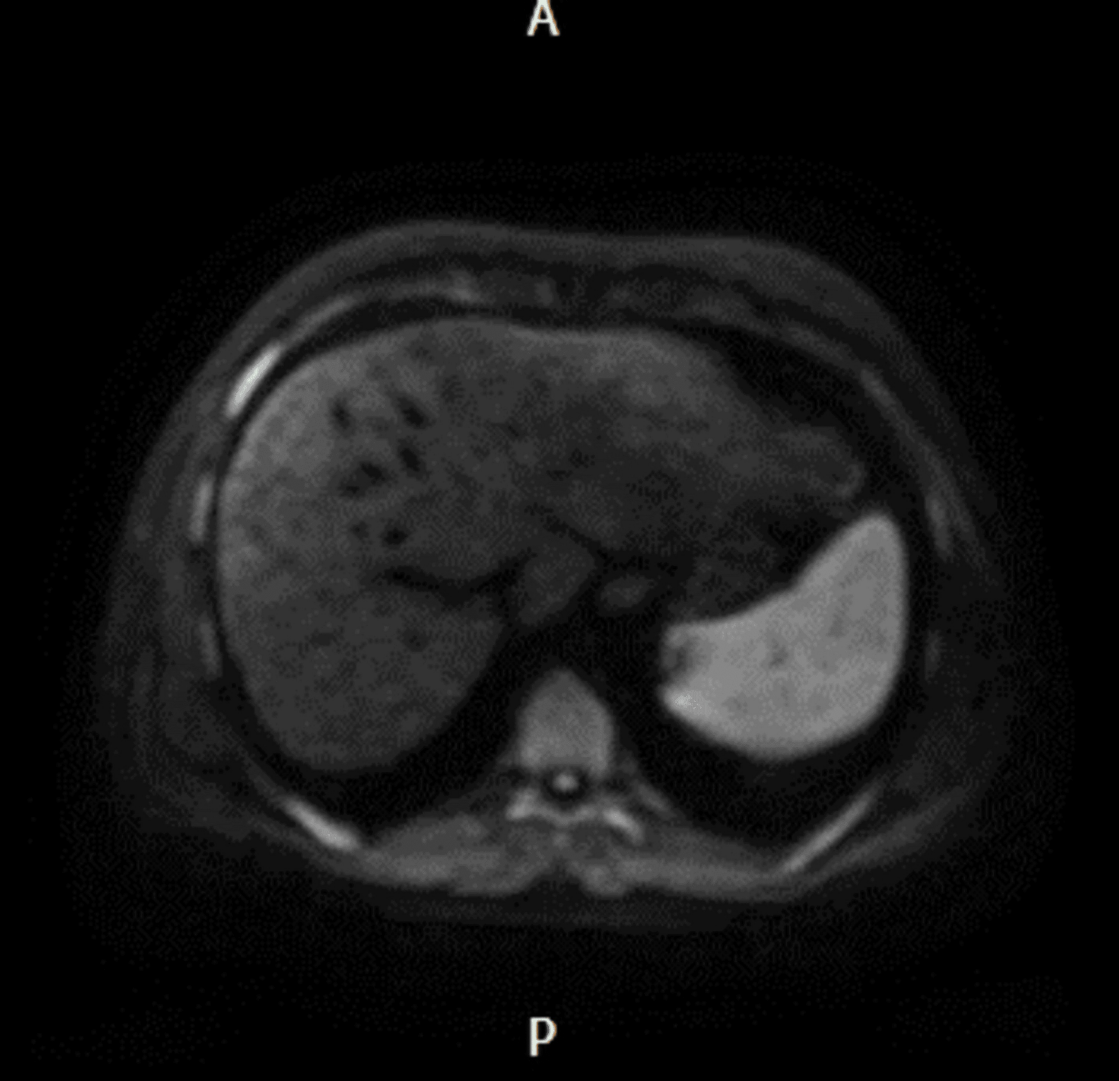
MRI showing an enlarged spleen and multiple enhancing lesions in the liver.
